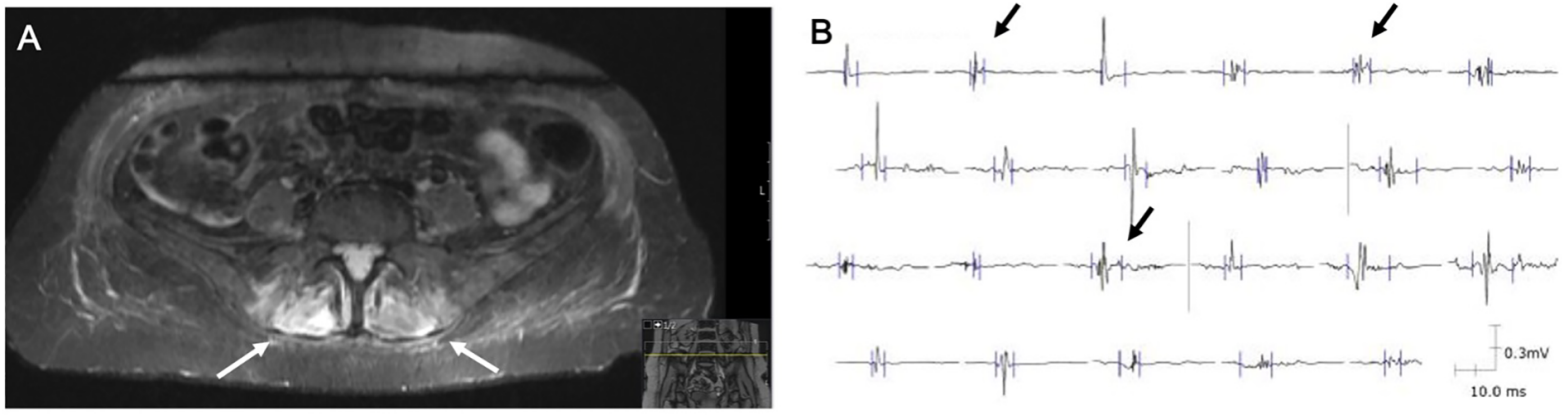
Radiological and electromyography findings leading to the diagnosis of ICI-induced myositis. (A) MRI in the TIRM sequence showing hyperintense signals in the autochthonous spinal muscles (white arrows). (B) EMG of the right gluteus medius muscle displaying polyphasic, short-duration, low-amplitude motor-unit potentials (black arrows).
